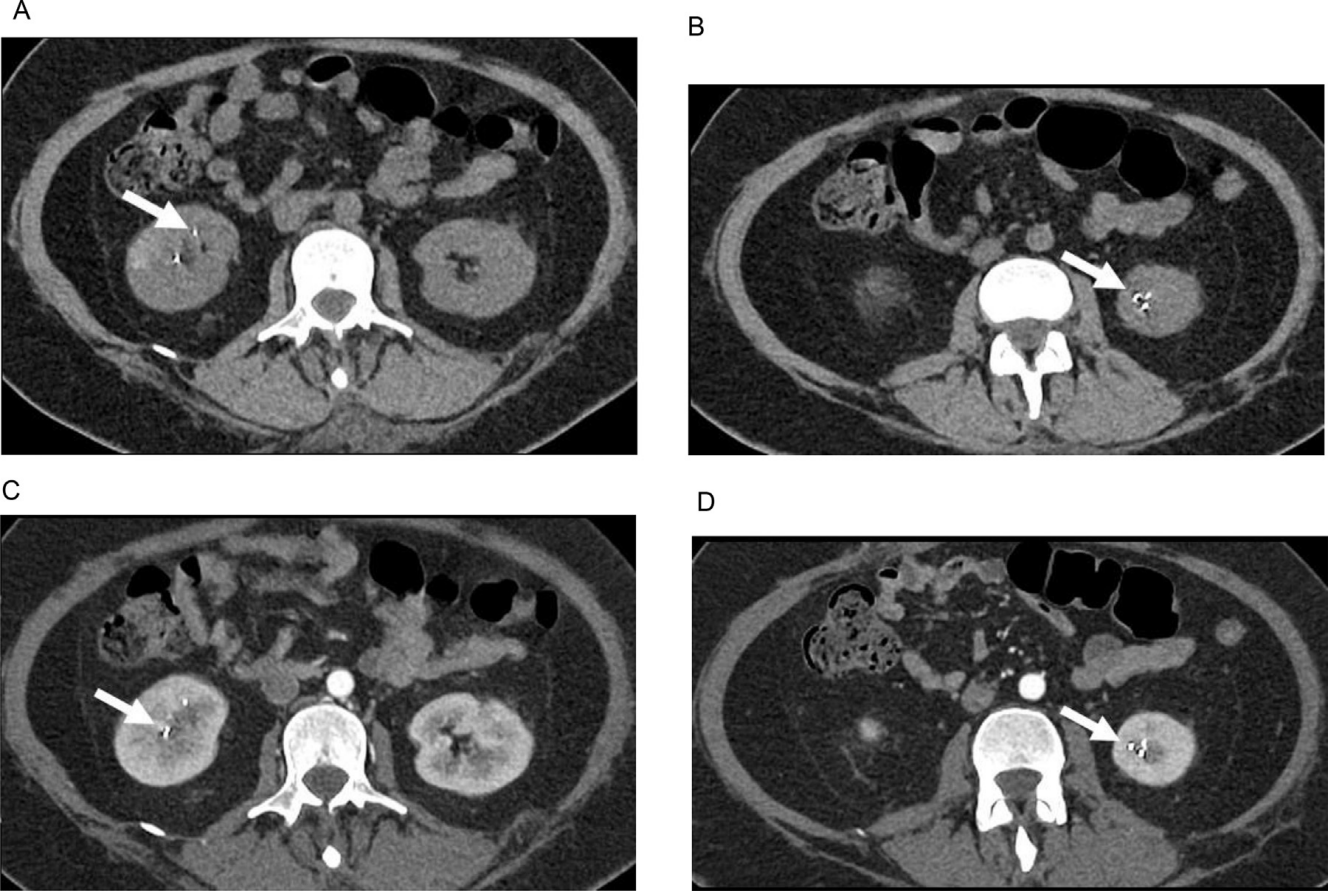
Abdominal axial CT scan in soft tissue window. (A and B) Unenhanced CT shows hyperdense foci in the inferior renal poles (arrows). (C and D) Enhanced CT revealed a relation of these foci with the renal arteries.
